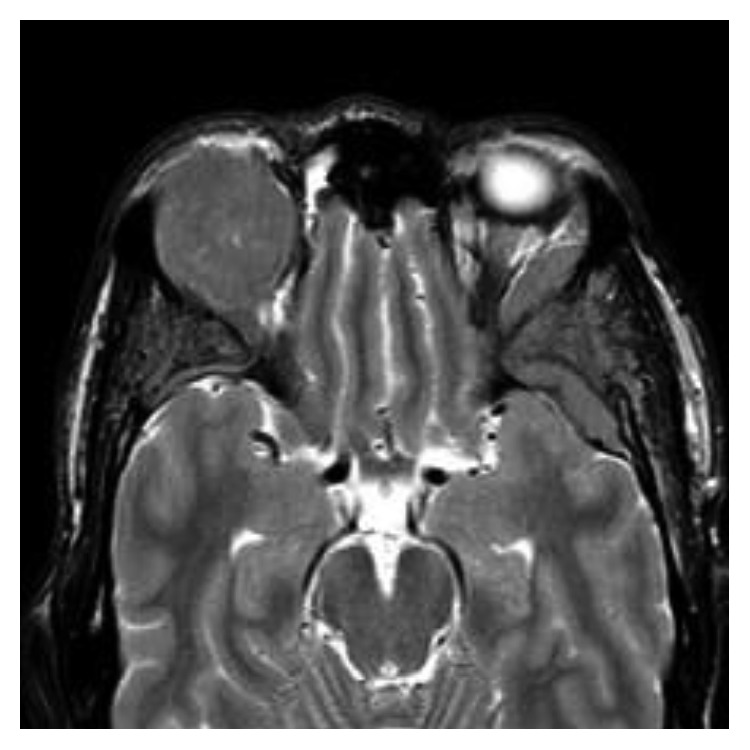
MRI before KRd treatment: increase in size of soft tissue in the right intraorbital region, pressing against the upper and lateral rectus muscle; the same soft tissue was observed in the extrassial site close to the large sphenoid wing, pressing against the left temporal muscle.
